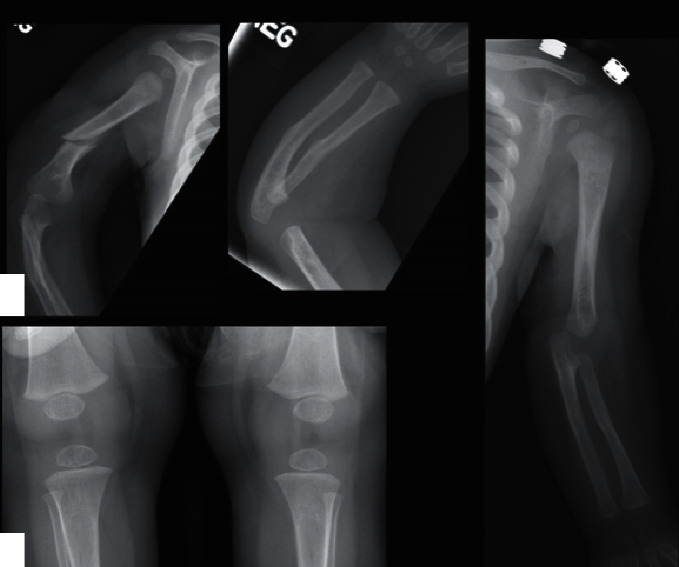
Spiral fracture of the right humerus is present (a). A right forearm radiograph shows healed midshaft fractures of the radius and ulna (b). The left upper extremity radiograph shows midshaft sclerosis and subtle cortical irregularity of the humerus, radius, and ulna, suggestive of healed fractures (c). The bone density is normal and no rarefaction of the zones of provisional calcification is present in the wrist and knees (b–d).
